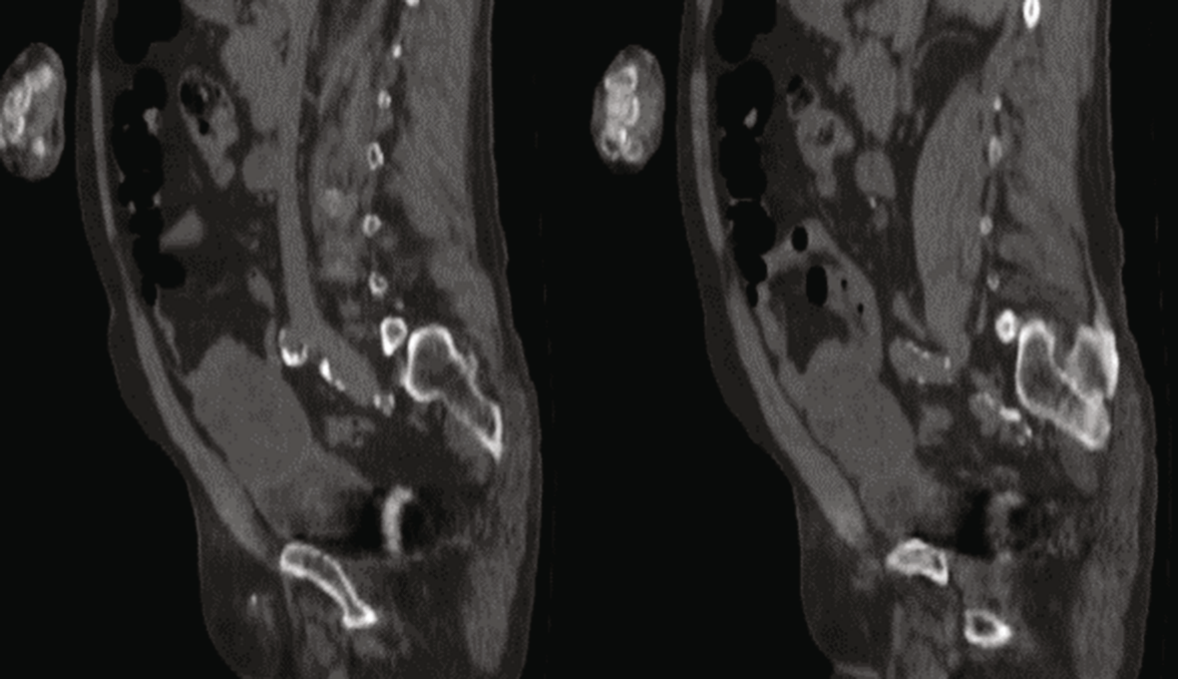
Sagittal cross-sectional imaging via CT abdomen and pelvis revealing an exophytic mass arising at the dome of the bladder.
